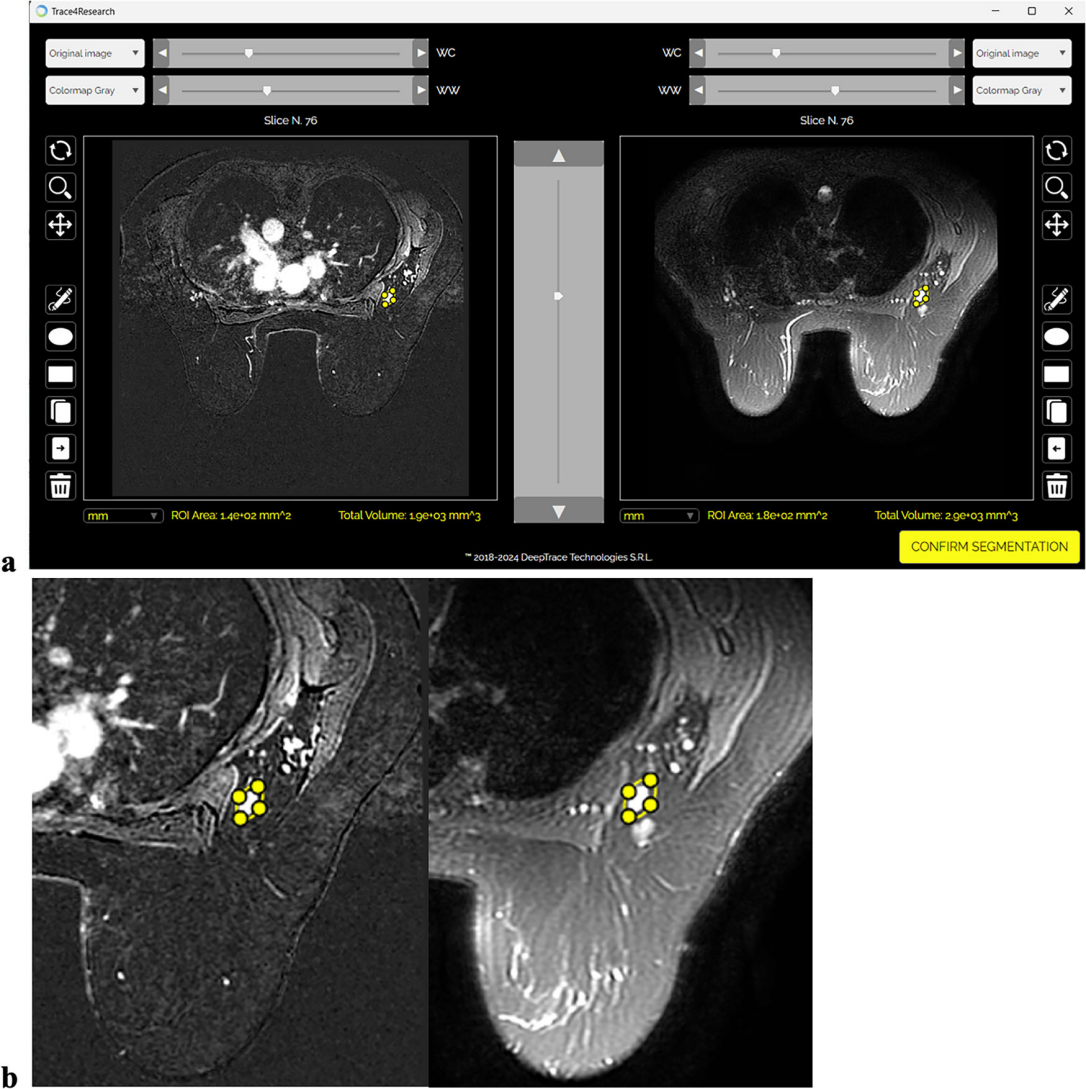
TRACE4Research™ platform: segmentation panel showing the contrast-enhanced subtracted images on the left and the T2-weighted images on the right (a); magnified left breast and axilla with node segmentation on both sequences (b)
